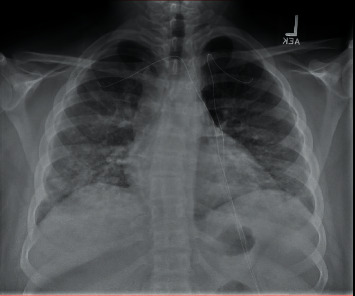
Chest X-ray showing interstitial opacities throughout the perihilar and basal lung regions.
